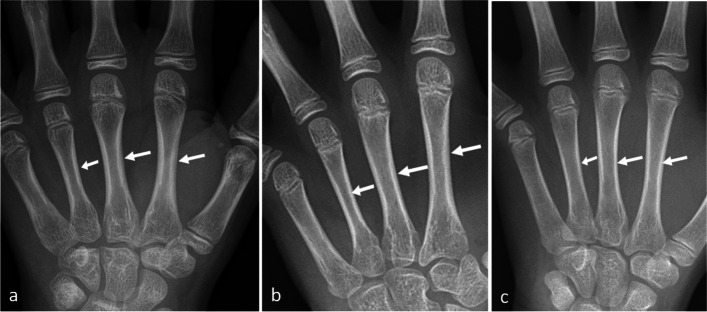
a-c Radiographs of the wrist in 3 females aged 10-11 years. Cortical thickness based on metacarpals 2-4 (arrows) was assessed using BoneXpert BHI Standard deviation score (SDS), classified on a 0-5 scale, exemplified by (a) SDS of +1.8, (b) SDS of 0 and (c) SDS of -2.4
